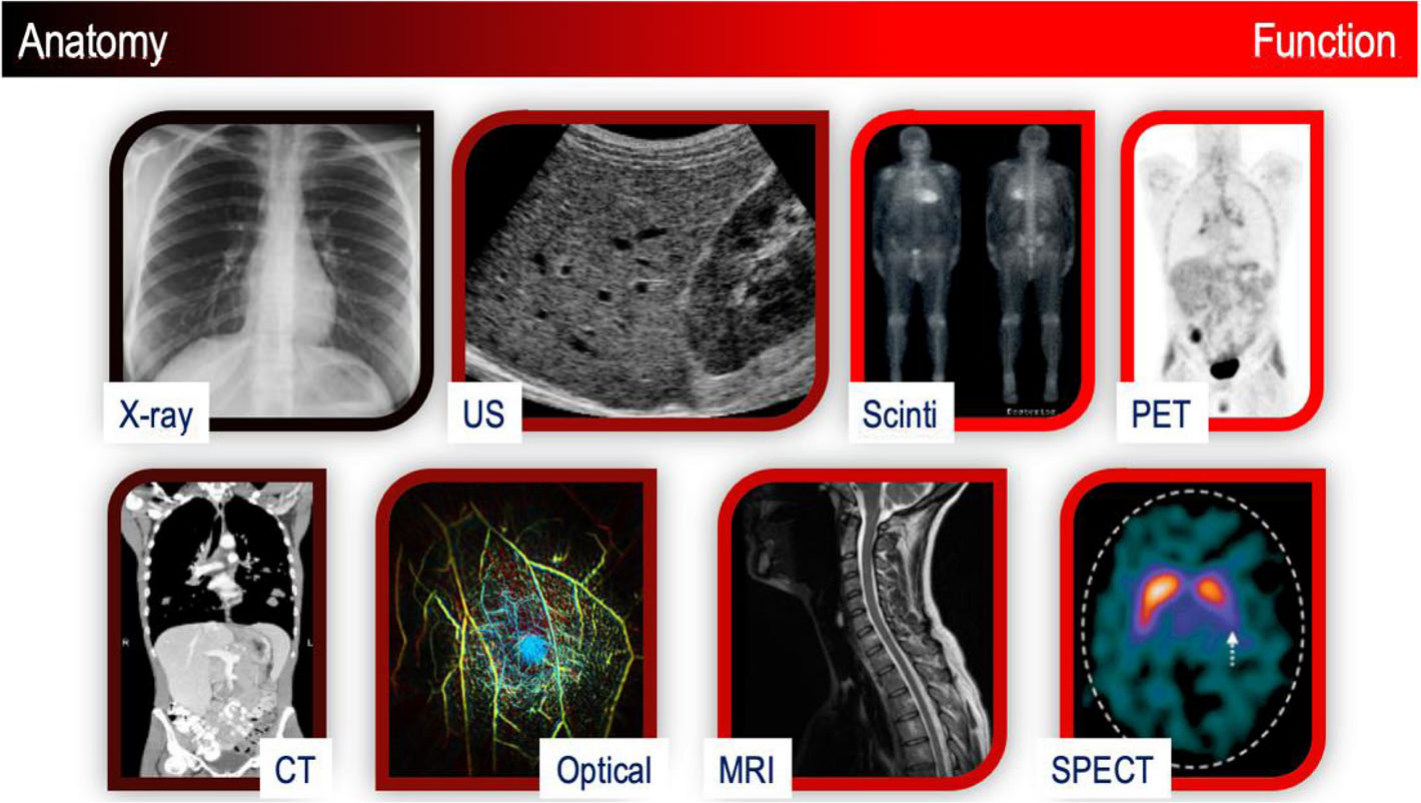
Imaging modalities together with their colour-coded ability to depict anatomical and/or functional information. For example, X-ray imaging is purely anatomical imaging modality, PET imaging provides functional (and molecular) data, while MRI and optical imaging are capable of providing anatomical and functional information depending on the choice of the protocol or mode of operation
